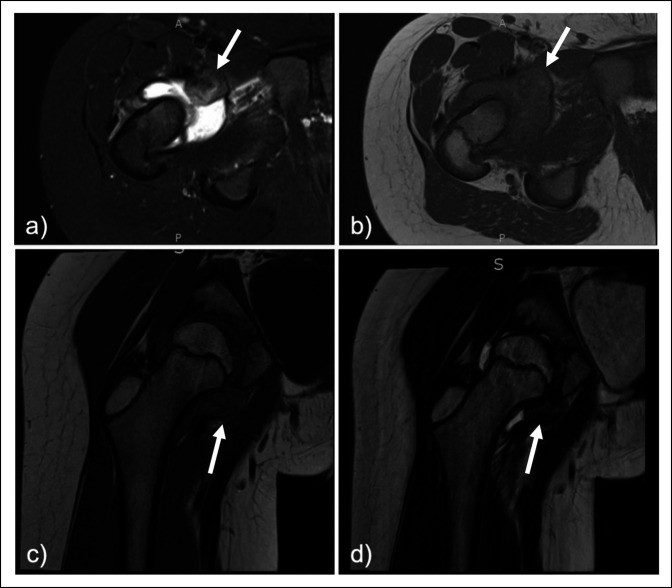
Radiographs showing short-time inversion recovery (STIR) axial (A), T1-weighted axial (B), T1-weighted coronal (C), and T2-weighted coronal (D) MR images of the hip demonstrating a 2.5 × 2.1 × 1.4 cm heterogenous mass within anterior-inferior joint space, with hypointensity in both T1 and T2 with enhancement (arrows). Heterogenous moderate right joint effusion with synovitis is also seen.
